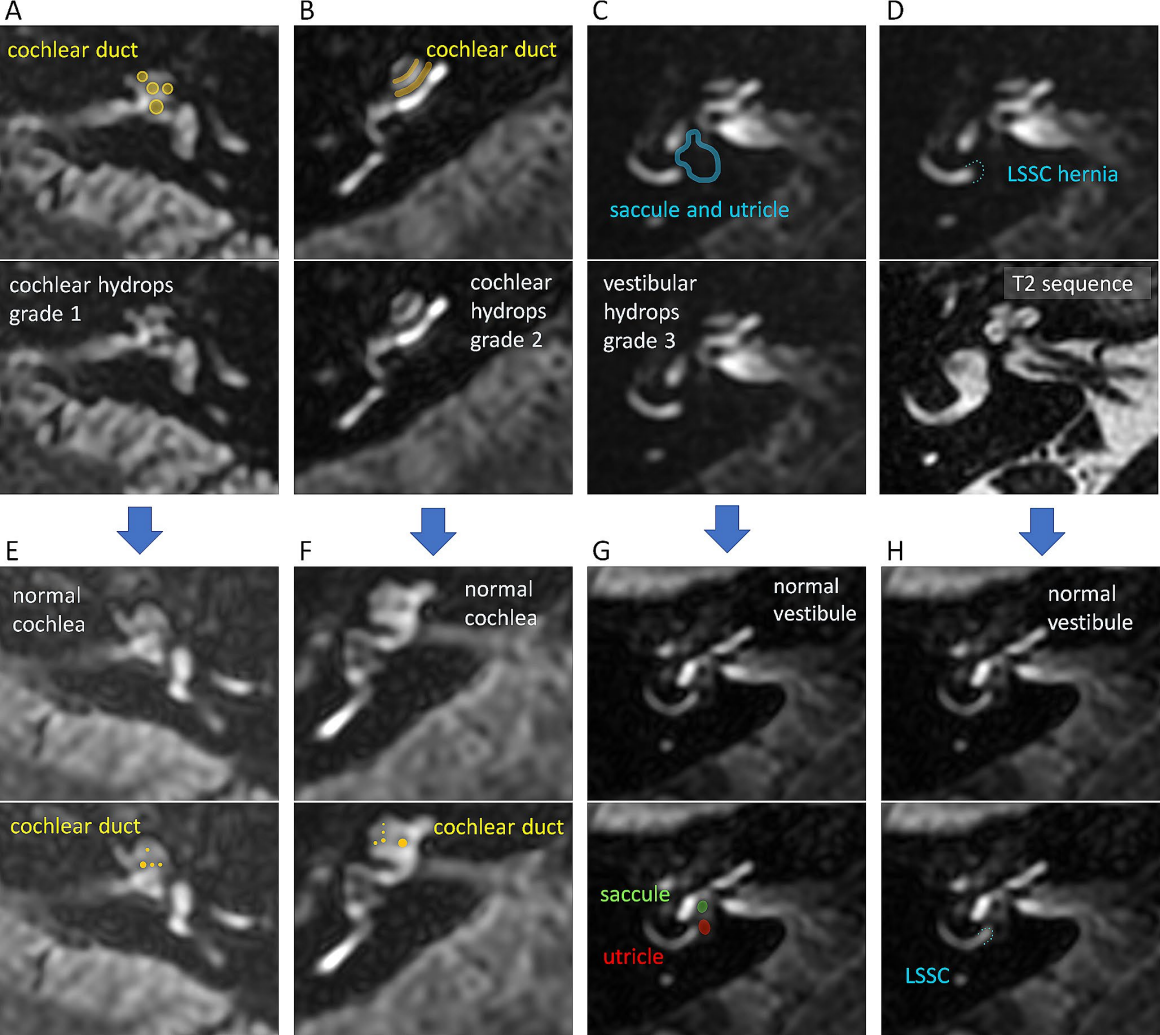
Magnetic resonance imaging (MRI) of patients with clinically diagnosed definite Ménière’s disease before and after vestibular neurectomy (axial plane, delayed Gd-enhanced images). Panels A-D show the preoperative MRI scans: (A) a dilated cochlear duct with partially obliterated scala vestibuli - grade 1 of the cochlear hydrops according to the Barath scale; (B) A dilated cochlear duct with fully obliterating scala vestibuli - grade 2 of the cochlear hydrops according to the Barath scale; (C) A dilated saccule and utricle occupying all the vestibule – vestibular hydrops grade 3 according to Bernaerts scale; and (D) Utricular herniation into the lateral semicircular canal (LSCC). 3D Heavily T2-weighted sequence visualizing preserved fluid signal. The upper images on panels A-D show the same images as the lower ones in respective panels but in the form of companion scans with some line art applied to define specific anatomical structures for easier identification of observed pathologies. Panels E-H show the postoperative MRI scans: (E) and (F) Complete regression of the cochlear endolymphatic hydrops, the narrow cochlear duct barely visible between the scala tympani and scala vestibuli; (G) Complete regression of the vestibular endolymphatic hydrops, saccule and utricle visualized as two separated, non-enhanced endolymphatic structures; (H) Withdrawal of the utrical hernia into the lateral semicircular canal (LSCC), the posterior arm of the LSCC confluent with the vestibular perilymphatic space. The bottom images on panels E-H show the same images as the upper ones in respective panels but in the form of companion scans with some line art applied to define specific anatomical structures for easier identification of observed pathologies
